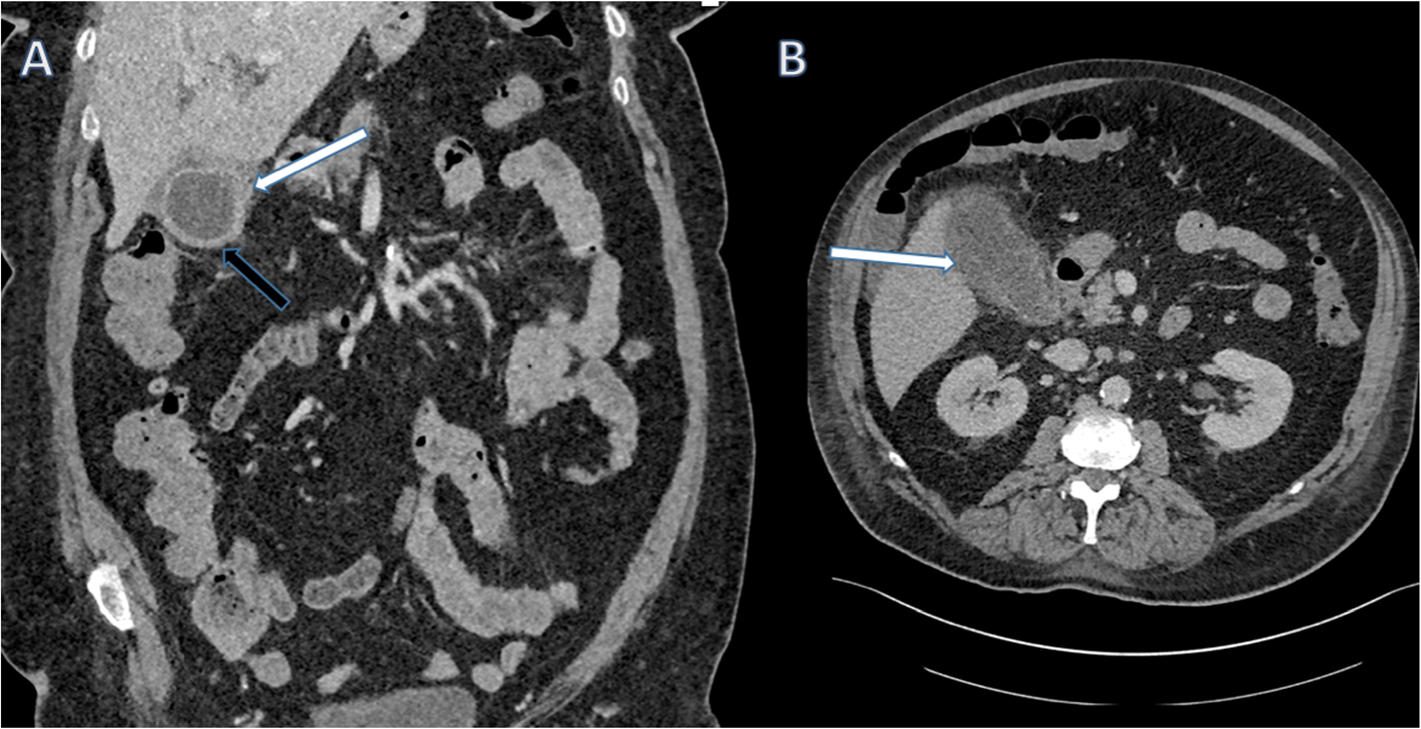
Coronal (a) and axial (b) contrast-enhanced CT of the abdomen. There is gallbladder wall thickening (black arrow) and pericholecystic fluid (white arrows) consistent with acute cholecystitis
